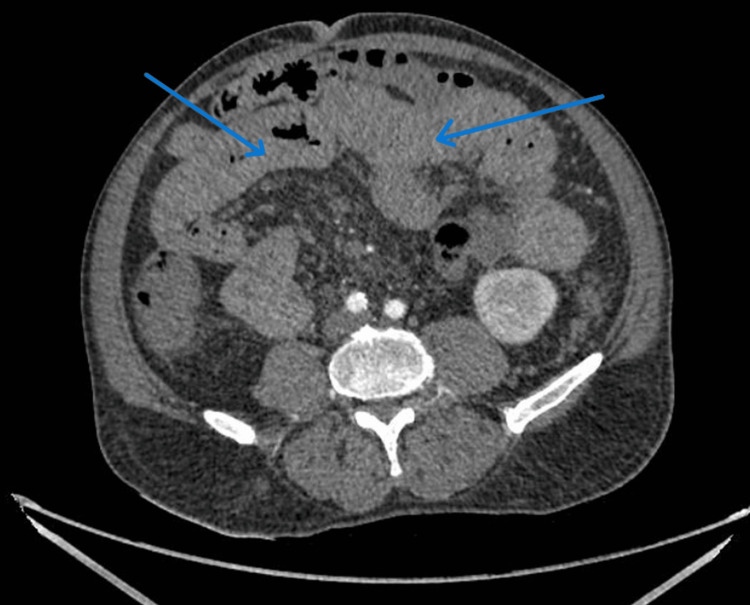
CT angiography of the abdomen. Blue arrows indicate the proximal colon and ileum, showing marked wall thickening, highly suspicious in the clinical context of arterial or venous ischaemia.
